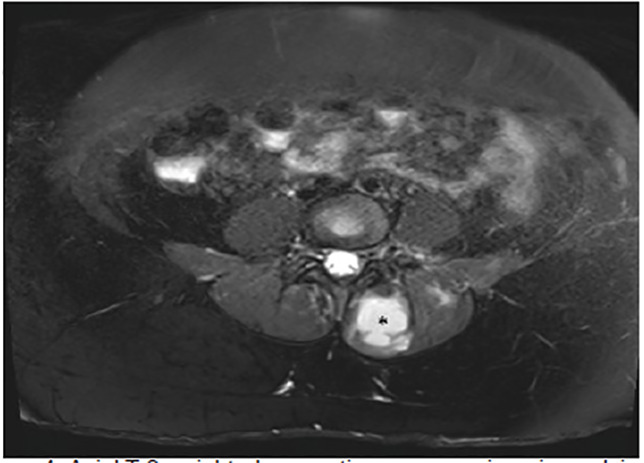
Axial T-2 weighted magnetic resonance imaging pelvis revealing a 3.4 x 3.2 x 7.9 centimeter abscess involving the left paraspinal muscle (*).
